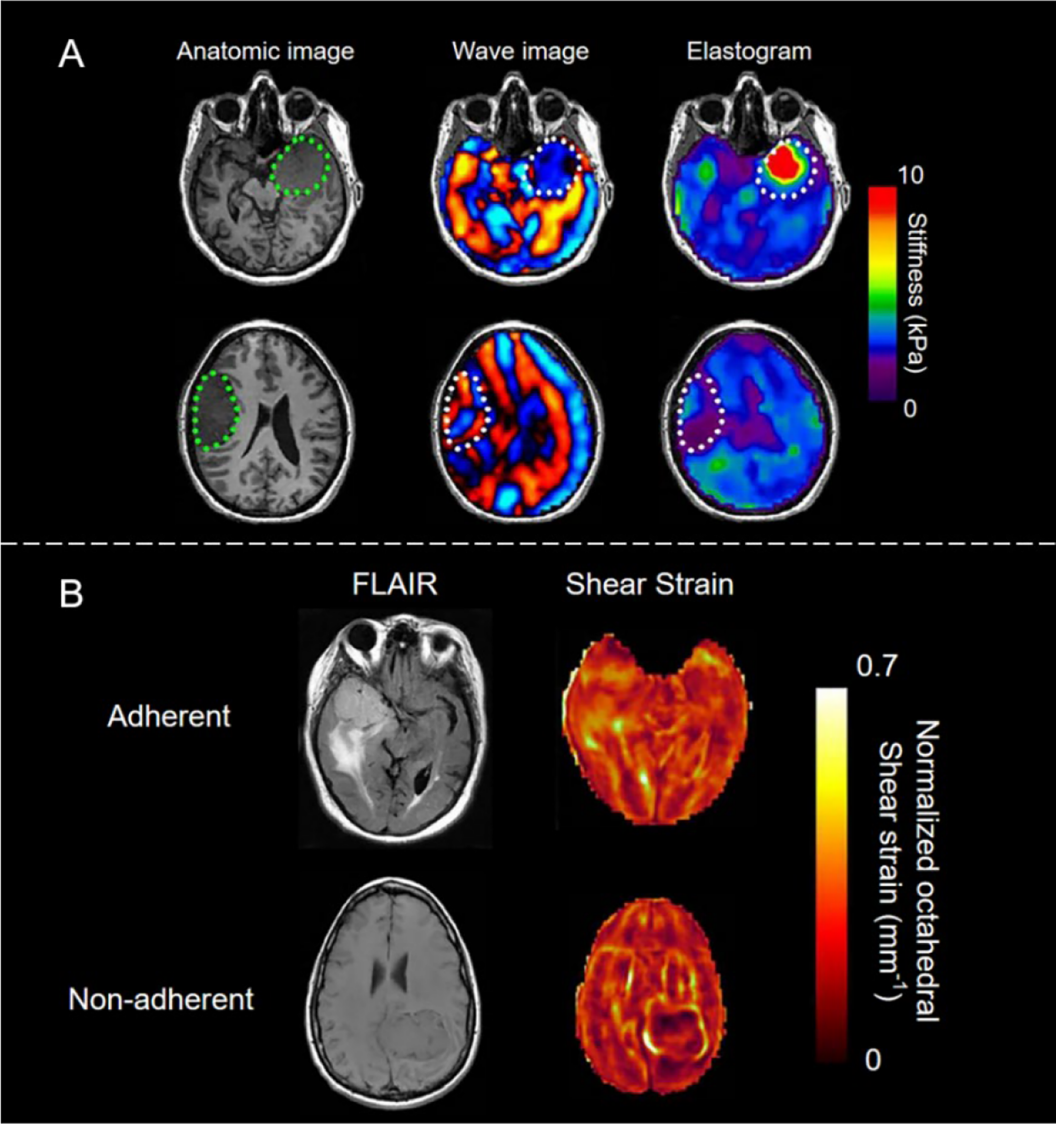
(A) Brain imaging of stiff meningioma subjects (top row) and soft meningioma subjects (bottom row). The area encircled by the green dots represents the location of the tumor. Normal brain parenchyma, dural meningioma, and soft meningioma have different mechanical properties, indicating the great potential of MRE in brain imaging. Reproduced with permission from ref. (62), copyright 2013, American Association of Neurological Surgeons. (B) The image of a sliding interface in the case of adherent (top) and non-adherent (bottom) meningiomas. In non-adherent cases, the tumor was surrounded by bright rings. Left: T2-weighted fluid-attenuated inversion recovery (FLAIR) images; Right: shear strain maps. Reproduced with permission from ref. (63).
